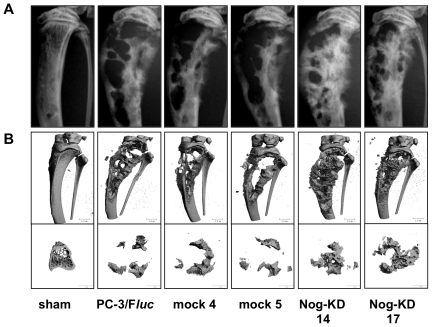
Noggin silencing promotes increase in radiodensity and partial bone repair in advanced osteolytic lesions. A. Representative images of radiography and B. 3-D reconstruction (µ-CT) of tibiae (top) and of 1 mm thick cross sections (bottom) of sham-operated and cancer cell-xenografted tibiae at day 25 after intra-osseous inoculation.
